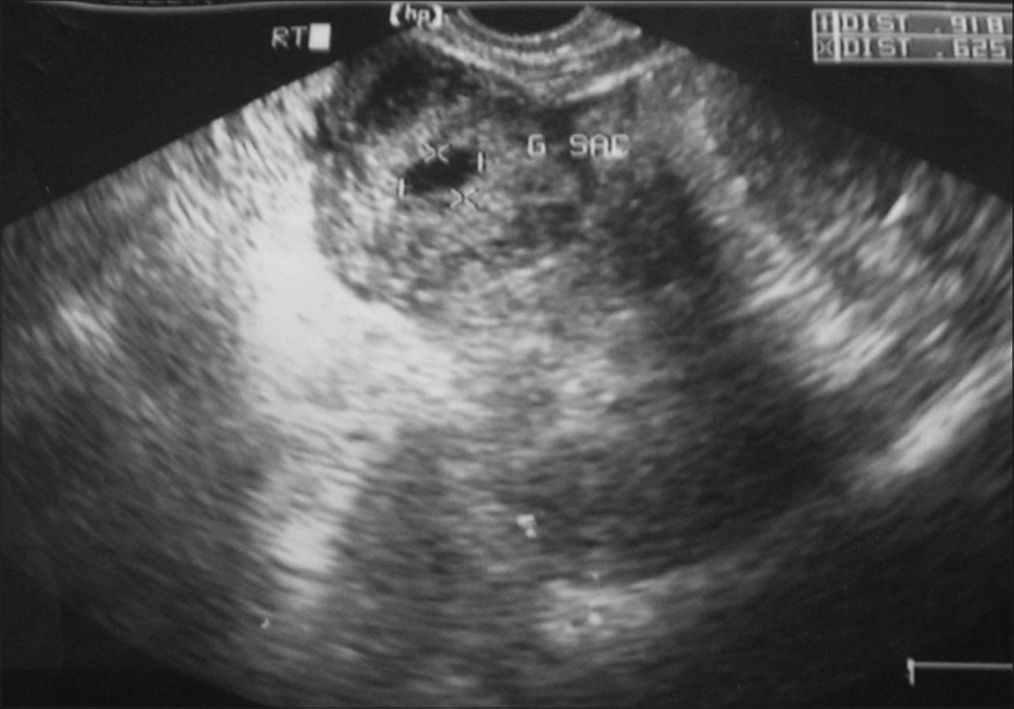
Ultrasonogram showing ectopic gestation
